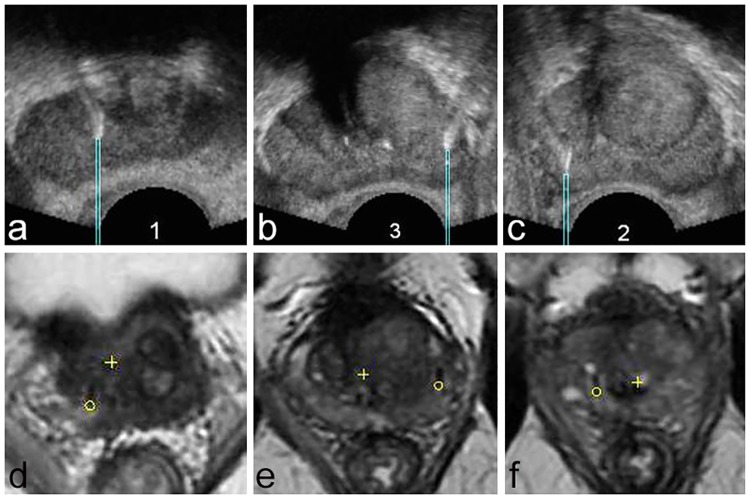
Images showing the three markers with virtual biopsies and MR targets (same patient as in Fig 1). A-C) Axial transrectal ultrasound images showing the markers implanted in the right base (A), the left mid-gland (B) and the right apex (C). A rectangular region of interest (‘virtual biopsy’) has been placed at the posterior extremity of each marker. This region of interest represents a virtual biopsy the tip of which would have reached the posterior extremity of the marker. The coordinates of the tip and base of the virtual biopsies were registered for each marker. D-F) Axial T2-weighted Turbo-Spin Echo images showing the markers implanted in the right base (D), the left mid-gland (E) and the right apex (F). A circular region of interest (‘MR target’) has been placed at the extremity of each marker. The coordinates of the center of these MR targets were registered for each marker. In case of perfect fusion, the tip of the virtual biopsies must correspond to the center of the MR targets.
